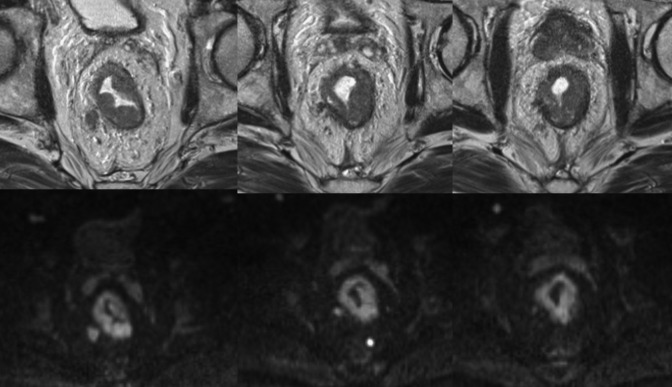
Areas of rectal cancer tissue on T2-weighted images (top row) corresponded to high signal intensity areas on diffusion-weighted images (bottom row) in a tumor estimated as T3a on pre-chemoradiotherapy magnetic resonance.
